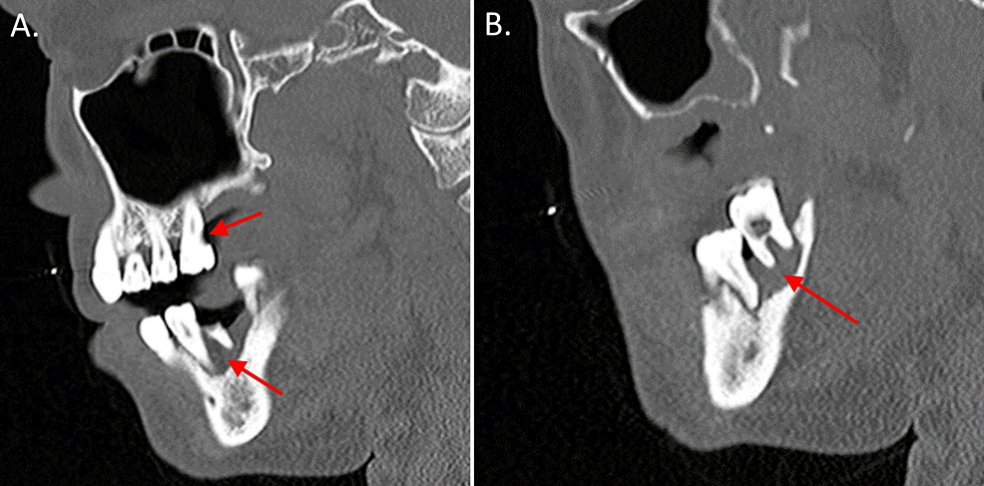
(A) CT scan of the maxillofacial area with contrast showing a sagittal view of the patient's endodontal and periodontal disease of the right teeth (arrows). (B) CT scan of the maxillofacial area with contrast showing a sagittal view of the patient's periodontal disease of the left teeth (arrow)
